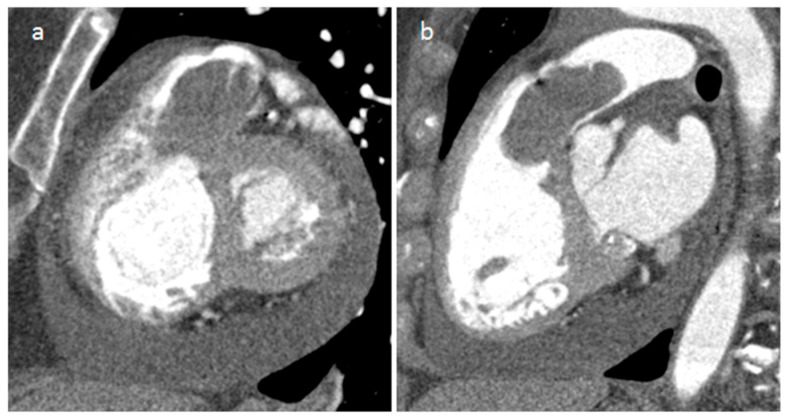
Right ventricular outflow tract and pulmonary primary cardiac leiomyosarcoma at CT (a,b).
